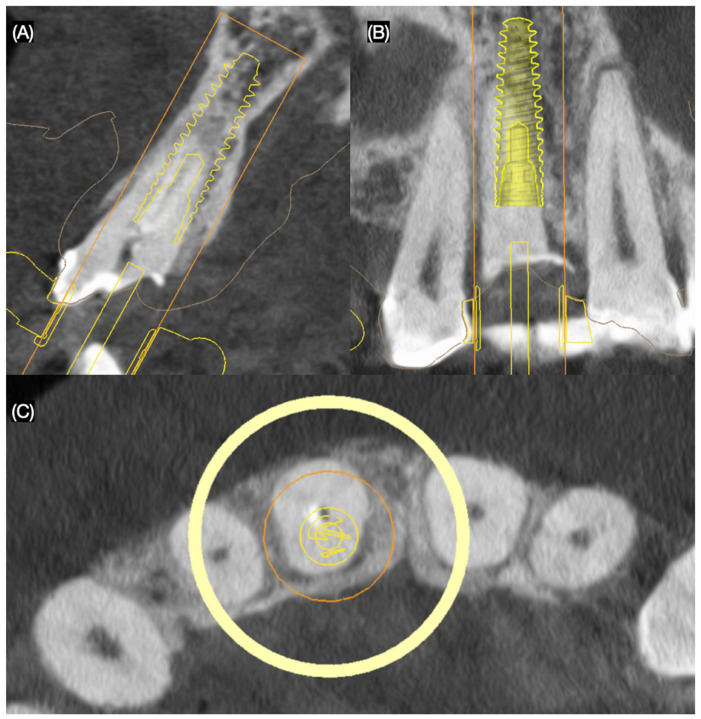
Three-dimensional implant evaluation with CBCT: (A) axial, (B) frontal, and (C) incisal view.
